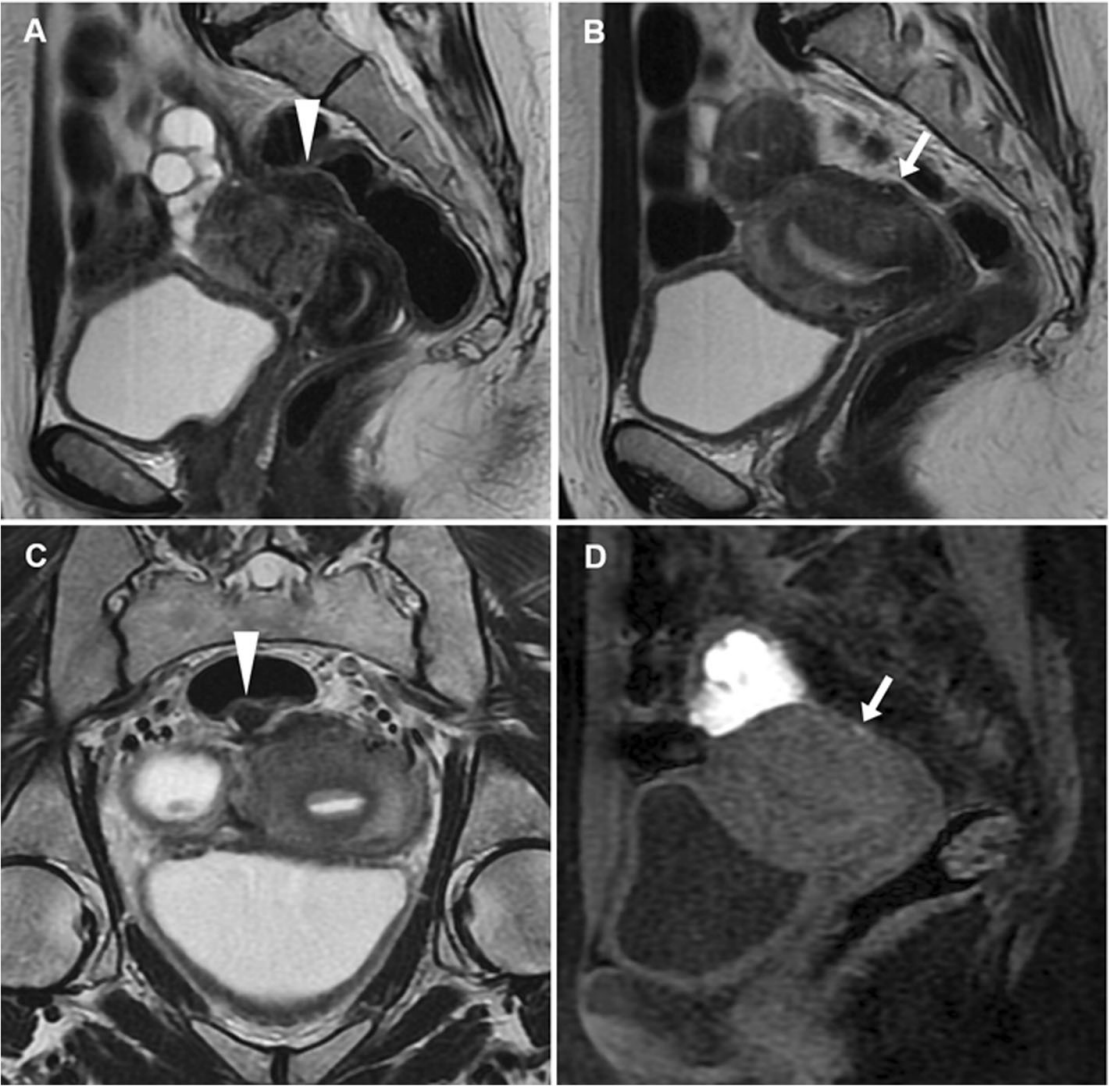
In the same patient as Fig. 16, sagittal (a) and oblique-axial (c) T2-weighted images show a hypointense endometriotic plaque (arrowheads) that infiltrates the muscular layer of the anterior rectal wall. Sagittal T2-weighted (b) and sagittal fat-suppressed T1-weighted (d) images show additional endometriotic implants of the uterine serosa (arrows) demonstrating low T2-weighted, high T1-weighted signal intensity
